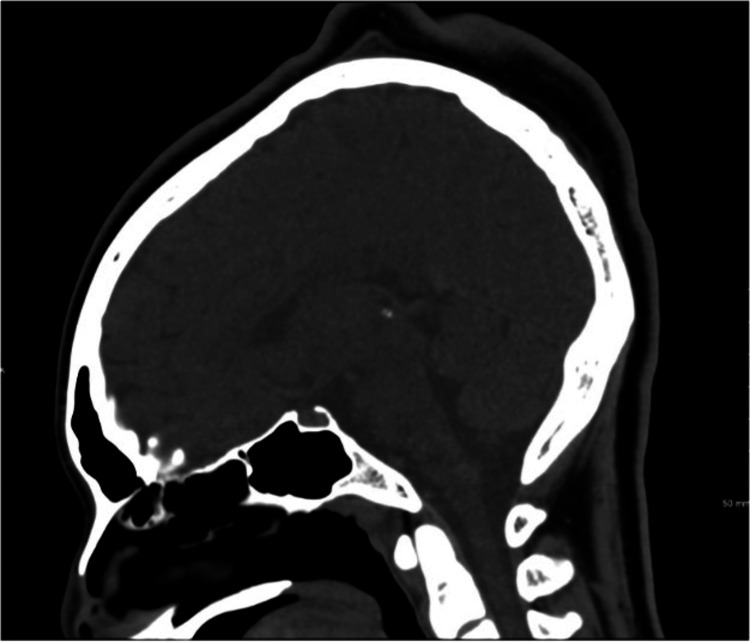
Sagittal noncontrast computed tomography of the head demonstrates an undulating contour of the scalp in the posterior parietal region
